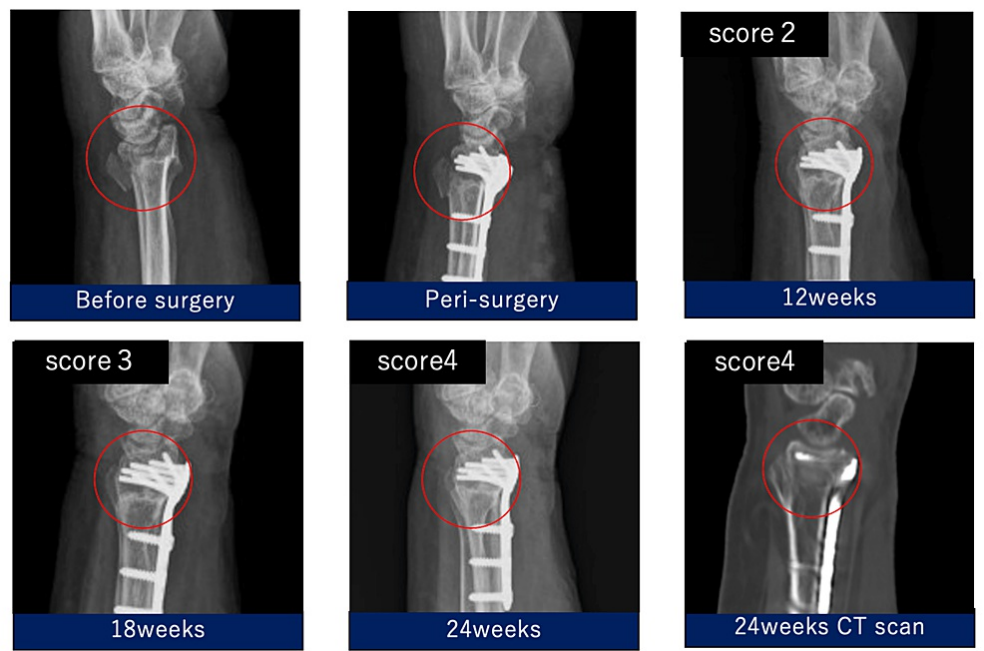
Radiographic evaluation of implanted ORB-03 for distal radius fracture. The circled area was filled with blood containing ORB-03 for the distal radius fracture and imaged preoperatively and at 12, 18, and 24 weeks after surgery.
